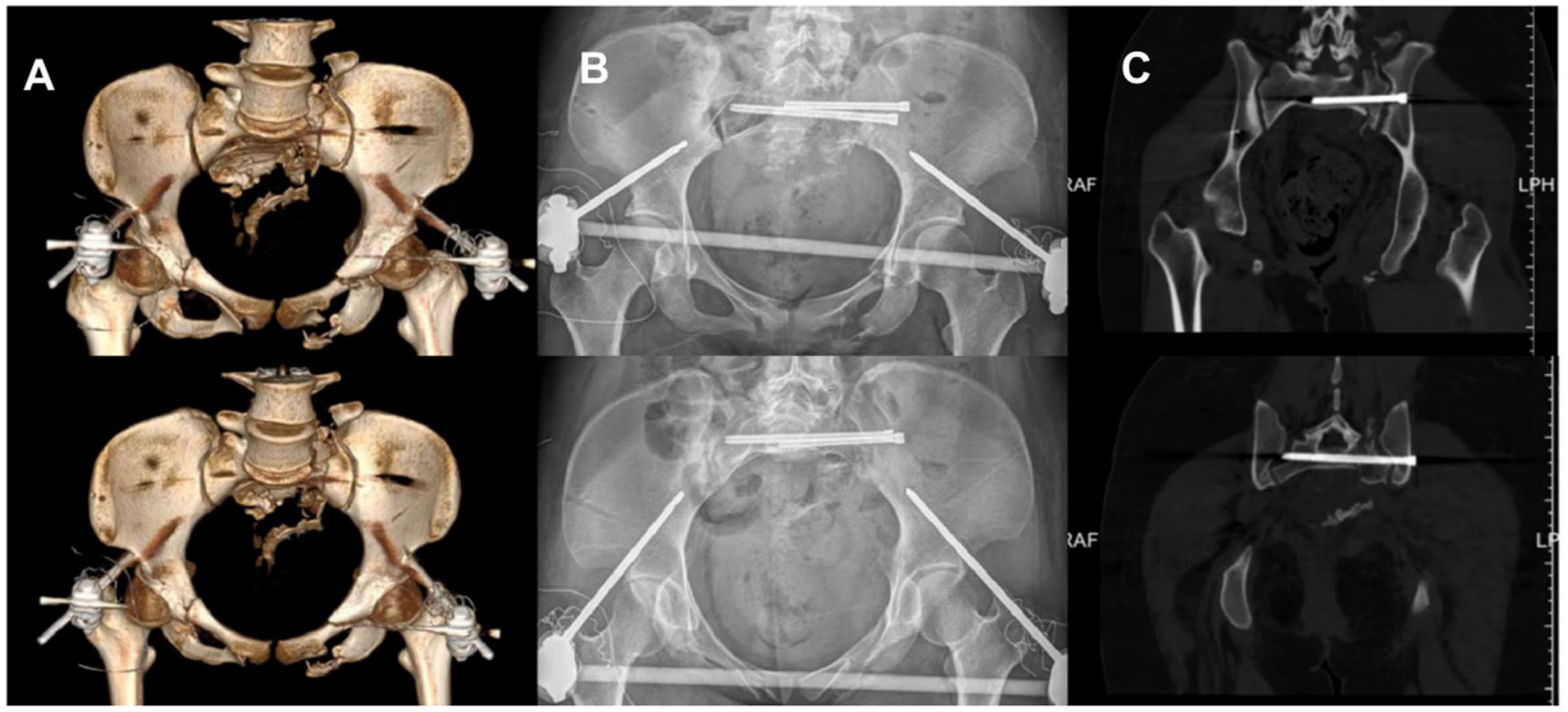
Postoperative X-ray and 3D CT images demonstrated the patient’s postoperative outcome. (A) Postoperative CT three dimensional reconstruction. (B) Postoperative pelvic X-ray orthopantomogram and entrance position. (C) Postoperative CT plain scan of the pelvis.
